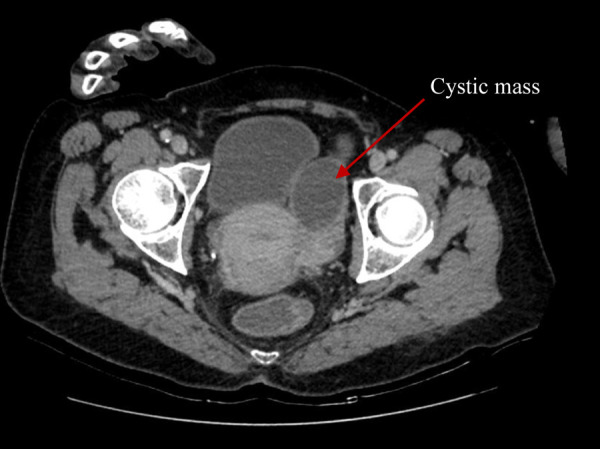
CT report of abdomen and pelvis. Cystic mass in the right adnexa of uterus (red arrow).
